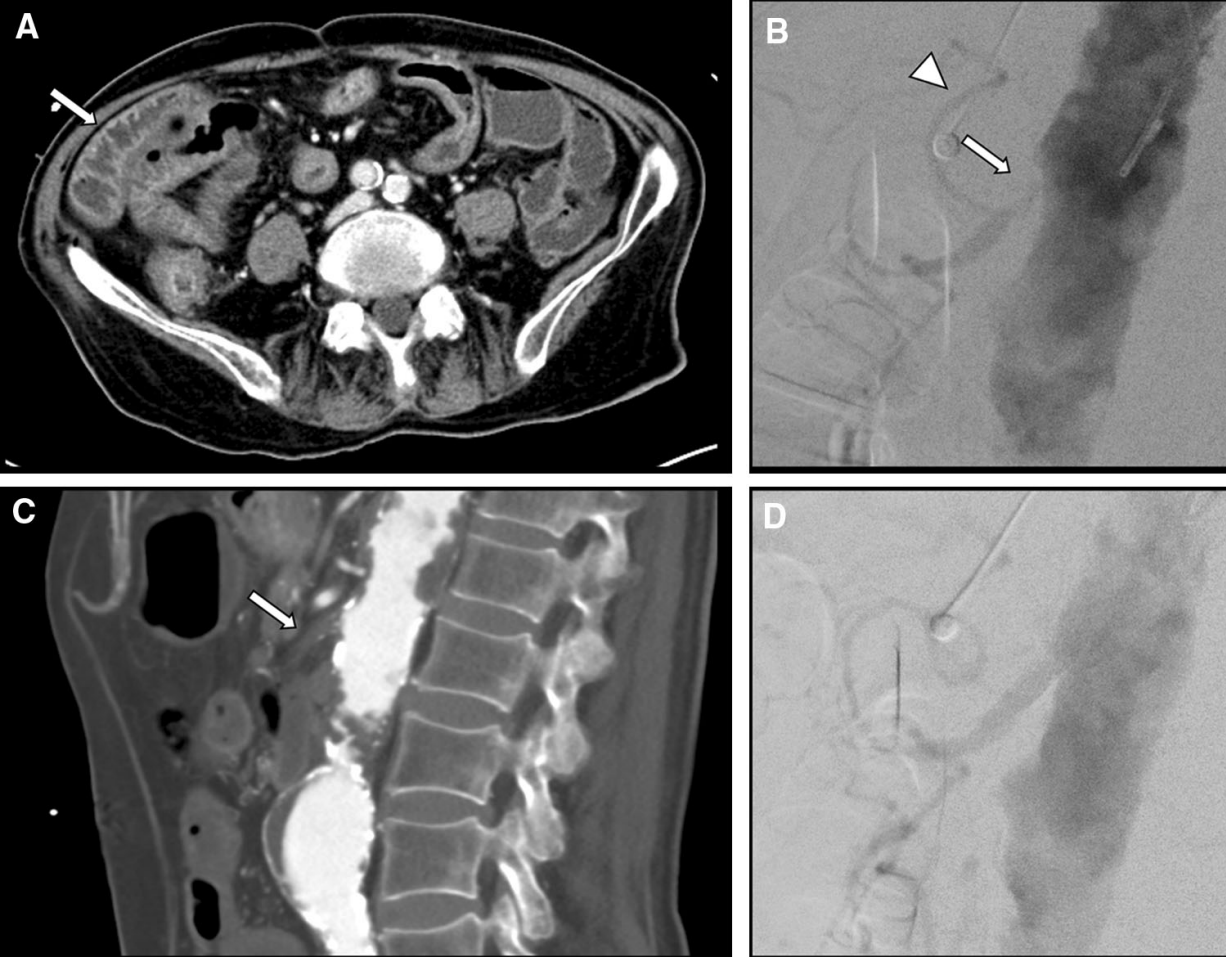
Sample case. 91-year-old male patient who presented to the emergency room because of acute worsening abdominal pain, as well as nausea and diarrhea. On the initial CT (A) edematous swelling of the bowel wall of the terminal ileum and right colon (arrow) was noted; the sagittal reconstruction of the arterial phase images showed a complete occlusion of the superior mesenteric artery at the origin resulting from arterial thrombosis due to severe atherosclerosis. The patient was immediately transferred to the angiography suite for emergency endovascular revascularization. Digital subtraction angiography confirmed the proximal occlusion of the superior mesenteric artery (B, arrow) with perfusion of the distal parts of the SMA and its branches via the gastroduodenal artery (arrowhead). Digital subtraction angiography after recanalization with stent implantation (6 × 16 mm Formula® 418 Vascular Balloon-Expandable Stent, Cook Medical, Bloomington) showed restored antegrade perfusion of the SMA (D)
